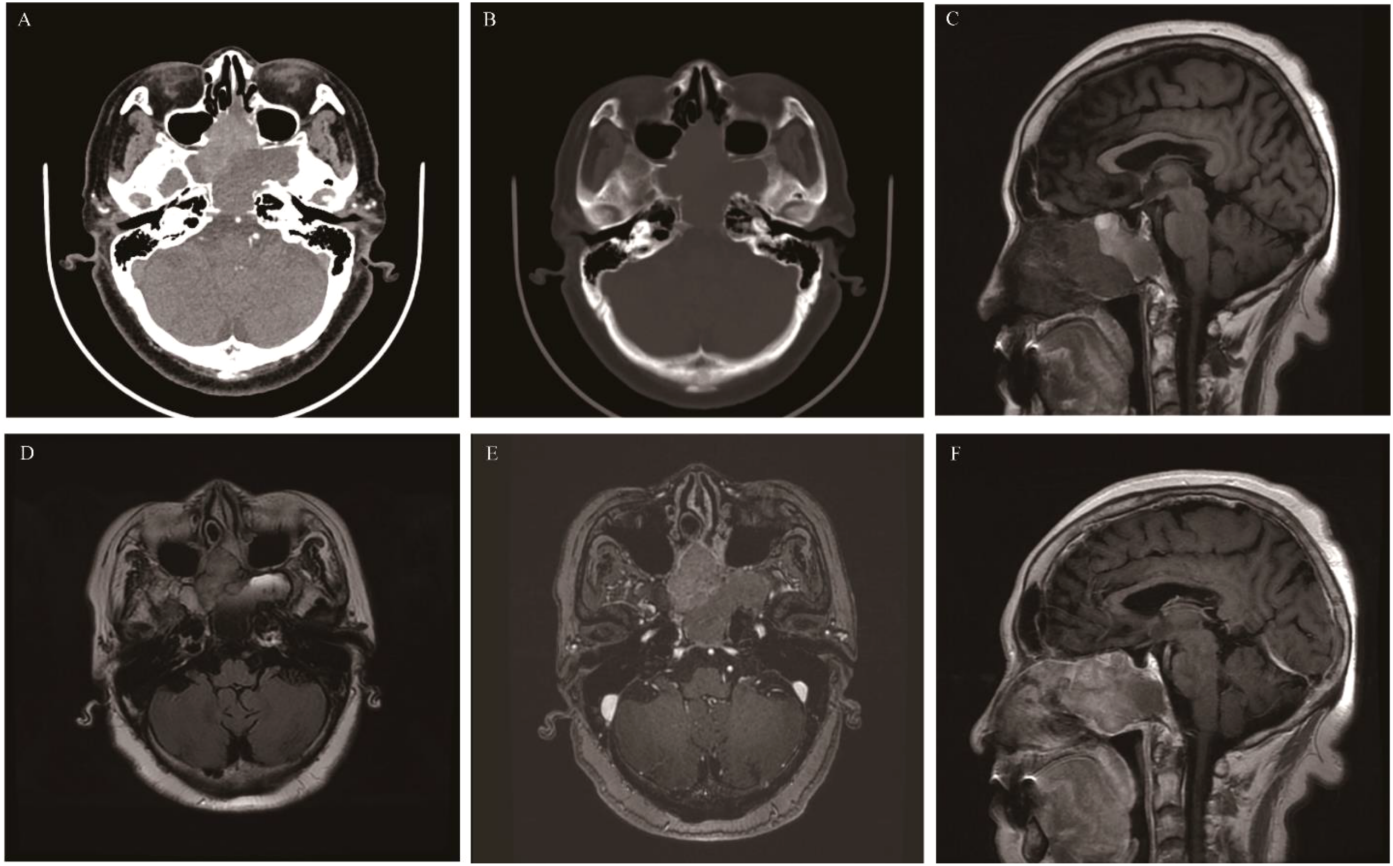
Sinonasal undifferentiated carcinoma: Axial CT showed the mass was mainly located at the top of the nasal cavity and involved the sphenoid sinus, ethmoid sinus, and clivus (A). The mass caused obvious bone destruction (B). It presented isointense on T1WI and isointense on T2WI, with heterogeneous enhancement on contrast-enhanced MRI On axial and sagittal (C-F).
